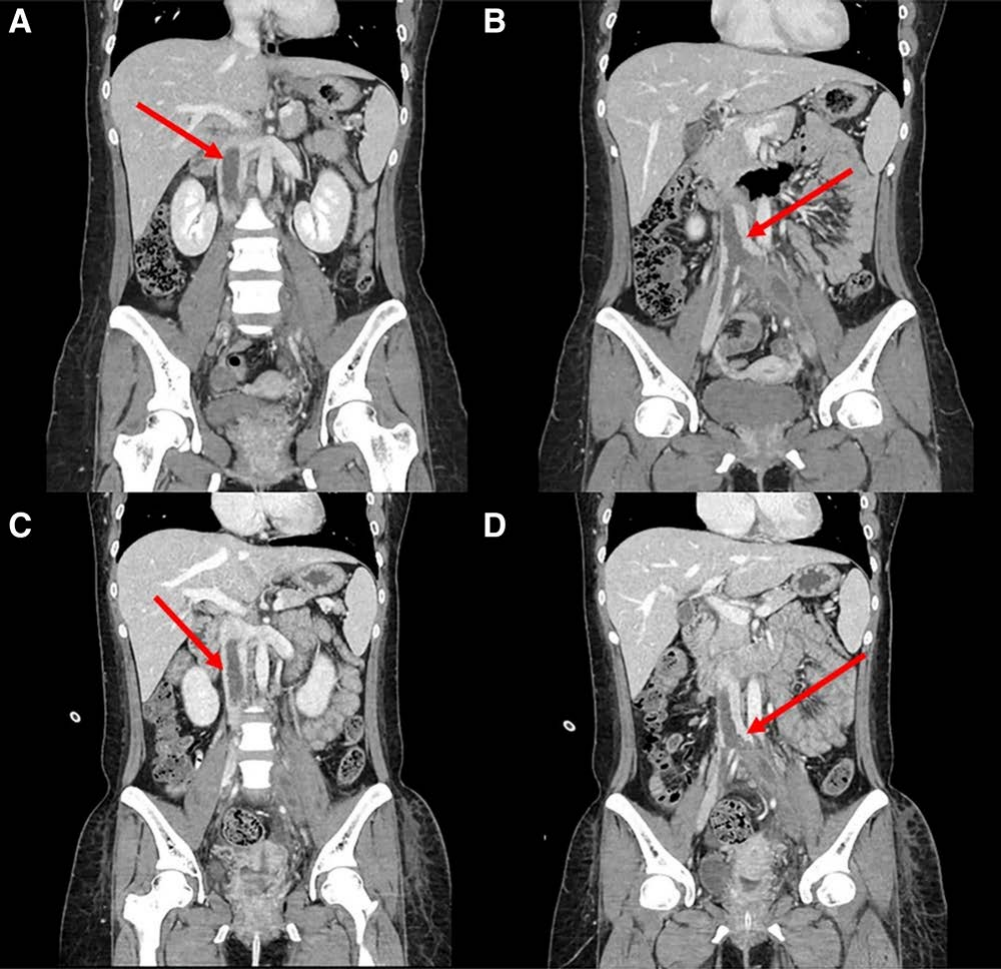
The initial abdominal CT shows a large IVC thrombus with a long segment. Red arrow lines show thrombus in the IVC. (A, B) CT scan was obtained before systemic thrombolysis. (C, D) CT scan was obtained 3 days after systemic thrombolysis. There was no significant change in the size of the thrombus before and after systemic thrombolysis. CT = computed tomography, IVC = inferior vena cava.
